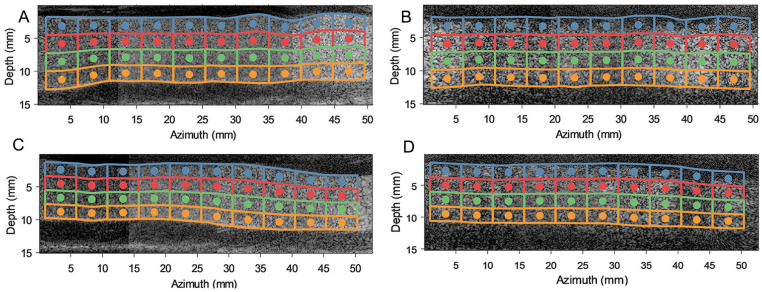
Before stenting and after stenting contrast-enhanced plane wave ultrasound images of the iliac arteries. The flow lumen is divided into 4 equally spaced vertical regions based on lumen’s centerline, with each region divided into 10 regions of interest (based on the average Anaconda ring distances). These regions are named “anterior wall” (blue windows), “anterior middle” (red windows), “posterior middle” (green windows), and “posterior wall” (orange windows). (A) Before endograft anatomy of left iliac artery. (B) After endograft anatomy of left iliac artery. (C) Before endograft anatomy of right iliac artery. (D) After endograft anatomy of right iliac artery.
